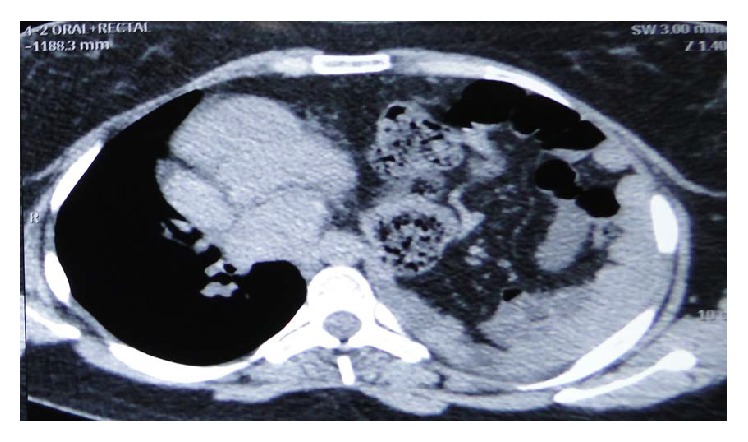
CT scan showing small intestine herniation in left Bochdalek hernia.
